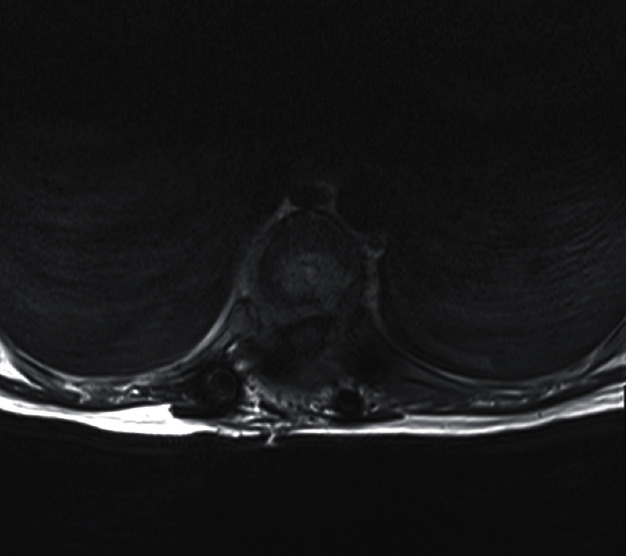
Postoperative MRI (axial image, showing the level of surgical decompression).
